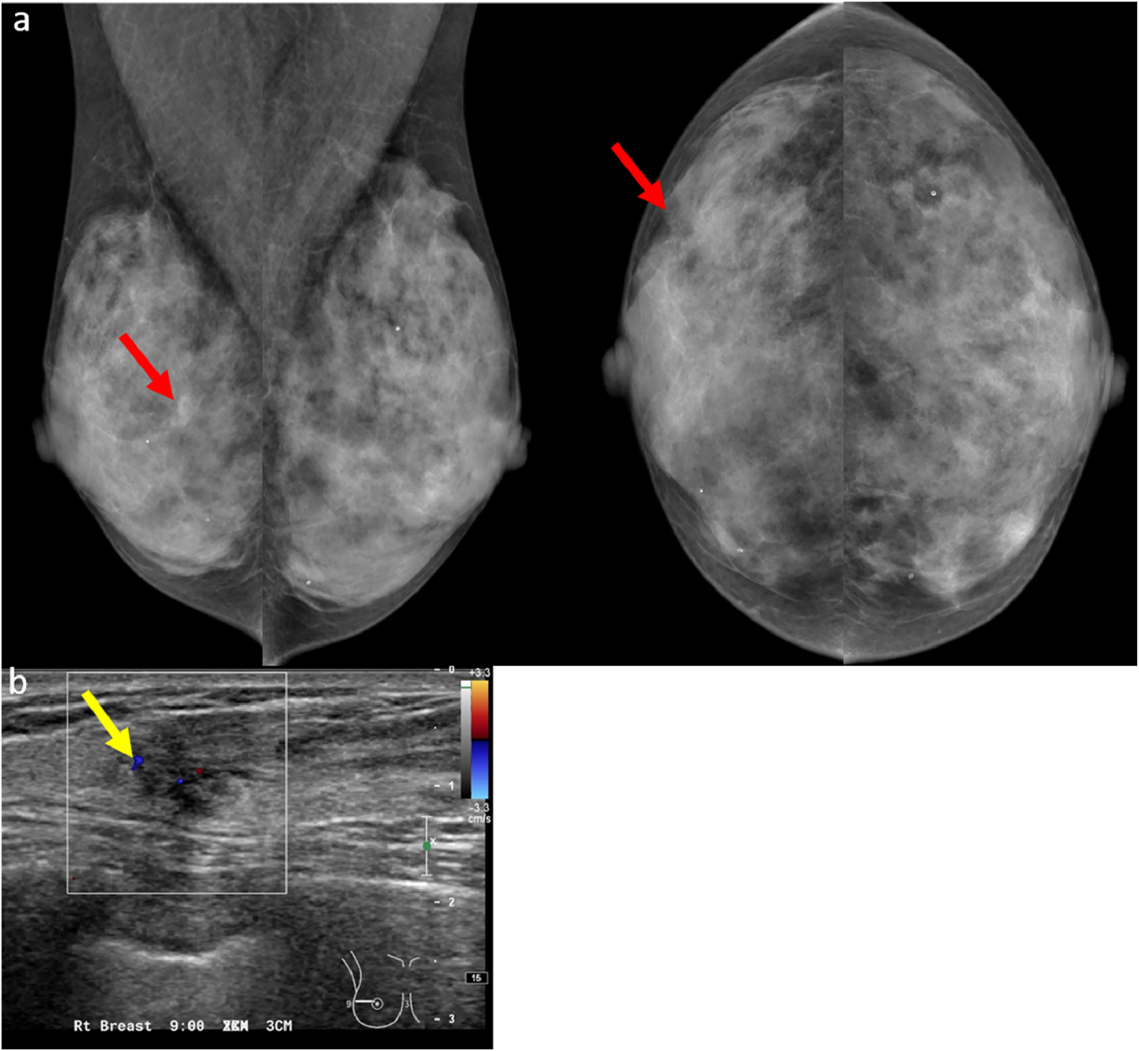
a First screening mammogram in a 43-year-old woman revealed a subtle architectural distortion in the outer central aspect of the right breast (red arrows) in otherwise extremely dense breast tissue. This finding was missed by one of the readers but detected by the second reader, who recalled the patient for further evaluation. Notably, there was a gentle retraction of tissue at the anterior mammary fat on the CC view, while the abnormality was difficult to appreciate on the MLO view due to overlapping dense breast tissue. b Targeted ultrasound revealed a correlating irregular hypoechoic mass in the same region (yellow arrow). Core biopsy confirmed the diagnosis of grade I invasive ductal carcinoma
